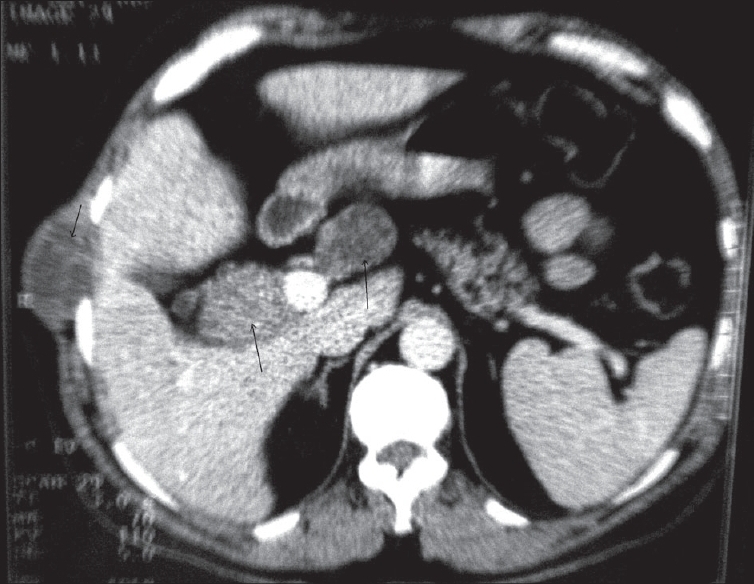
Contrast-enhanced axial CT image showing neurofibromas at the porta hepatis, peripancreatic region and right lateral abdominal wall
